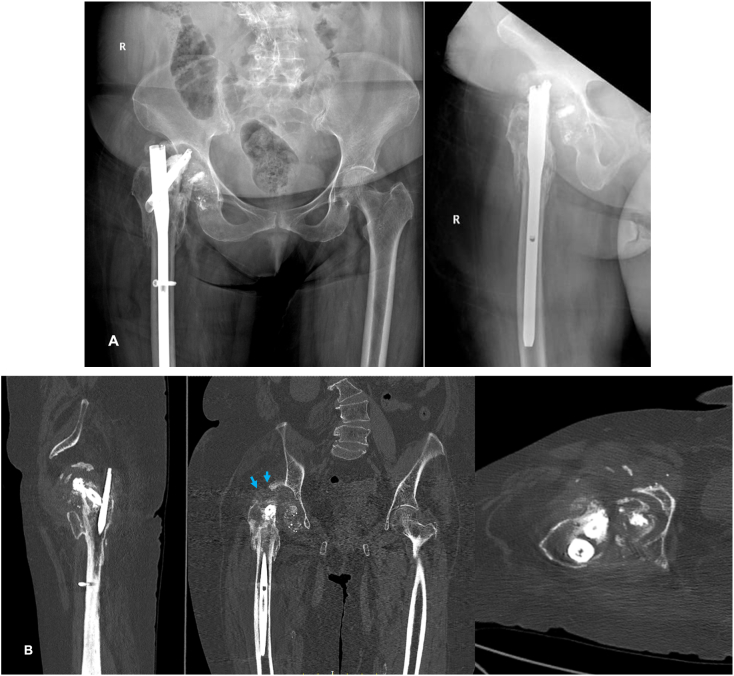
A) Cut through of helical blade – X ray hip Ap and lateral views at 23rd month follow up visit. B) CT Sections Showing ONFH, Cut Through of femoral blade and acetabular erosions (Blue arrow).
